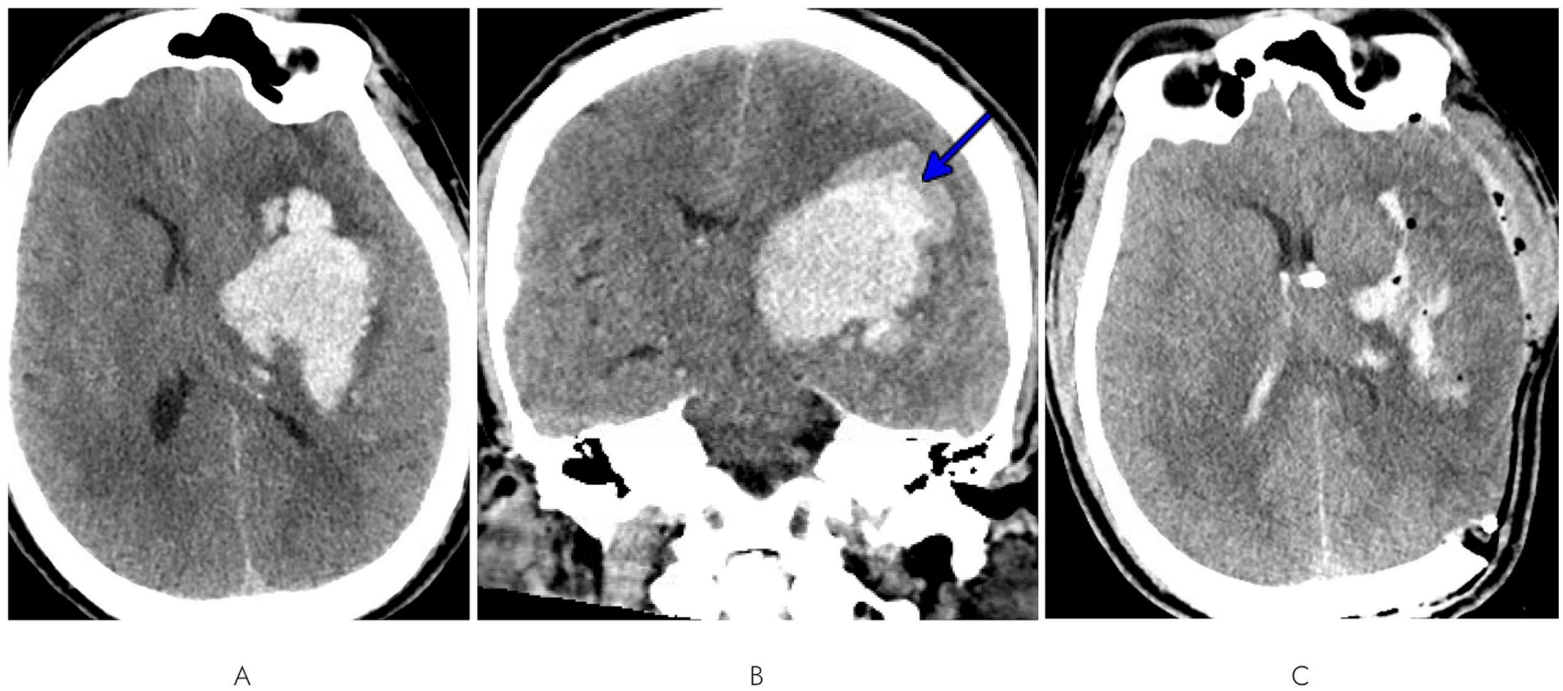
(A) Axial and (B) coronal views of initial CT head demonstrating a large left basal ganglia intraparenchymal hemorrhage with edema, subfalcine herniation, midline shift, and blend sign (blue arrow). (C) Post-craniectomy and EVD CT head displaying hematoma evacuation and corrected midline shift.
